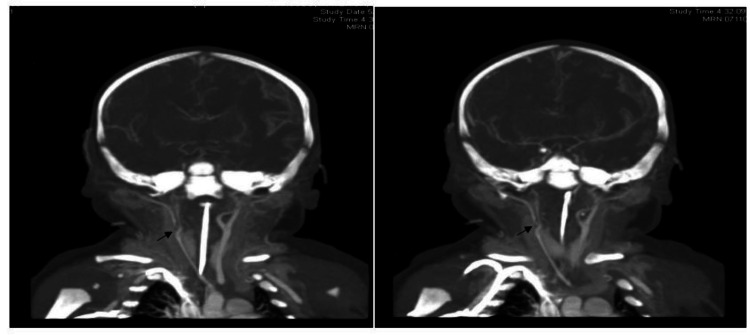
Computerized tomography (CT) angiography findings (Left, right) CT angiography demonstrated diffusely small caliber of the right common carotid and internal carotid arteries. Focal filling defect at the carotid bifurcation, thrombus or dissection that is being shown by the arrows.
